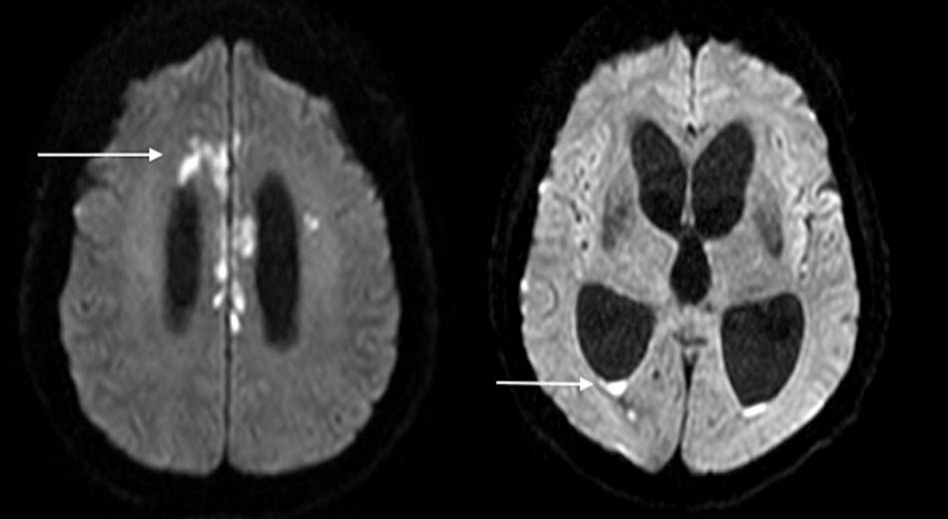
Diffusion-weighted MRI-brain showing intensely high signal in cerebral cortex (arrow) and ventricles (arrow) consistent with infective cerebritis and ventriculitis.
